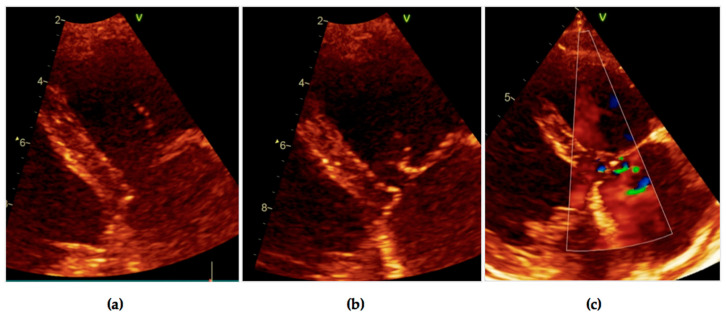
Transthoracic echocardiography, modified parasternal long axis–posterior leaflet of the tricuspid valve with chordal rupture, minor tricuspid regurgitation. (a,b) posterior leaflet of the tricuspid valve with chordal rupture; (c) minor tricuspid regurgitation.
